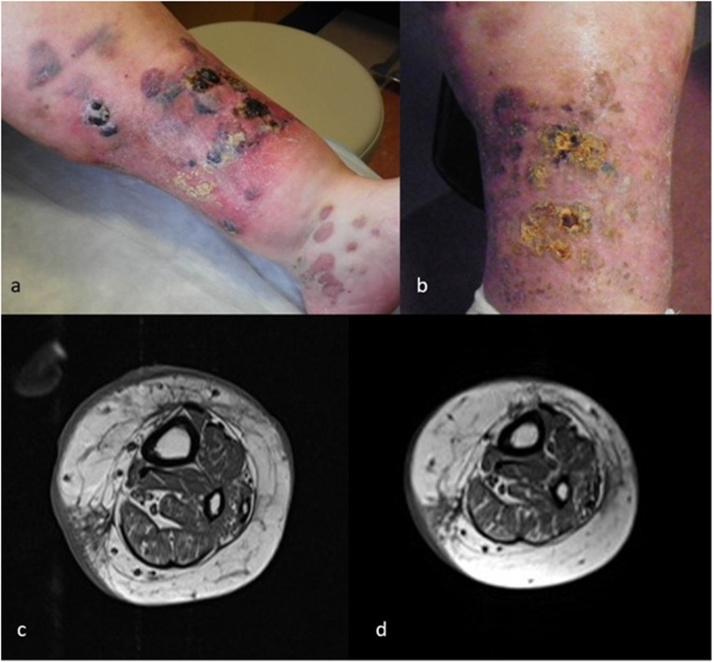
(a) AS of the left lower extremity at first presentation. (b) AS of the left lower extremity after chemotherapy. (c) MRI of left lower extremity angiosarcoma at first presentation. (d) MRI after chemotherapy.
